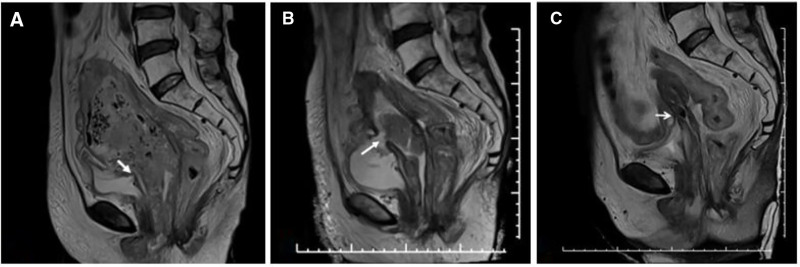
MRI of carcinoma invading reproductive organs. (A) Uterus, cervix and vargin were no longer visible. Bladder was partially invaded. White arrow indicated the lesion-blocked MVF; (B) after 4 cycle systematic treatment, tumor partially shrank. White arrow indicated the MVF was partially blocked, with signs of urine discharging from the vagina; (C) after 6 cycle systematic treatment, the tumor shrank significantly. White arrow indicated the MVF was no longer obstructed. MRI = magnetic resonance imaging, MVF = malignant vesicovaginal fistula.
